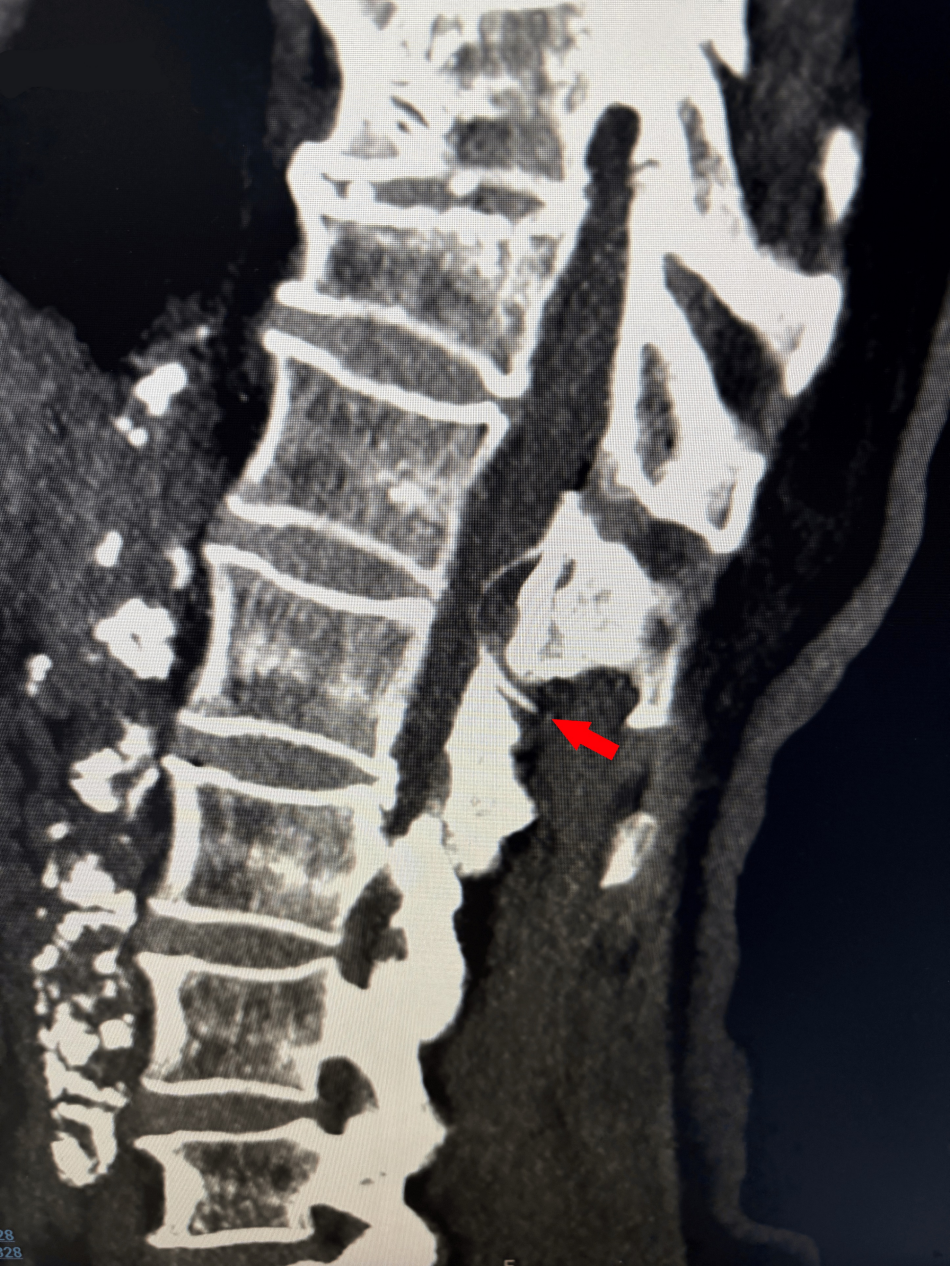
Spinal CT scan showing a fragment of the epidural catheter at the T12-L1 level. CT: computed tomography
